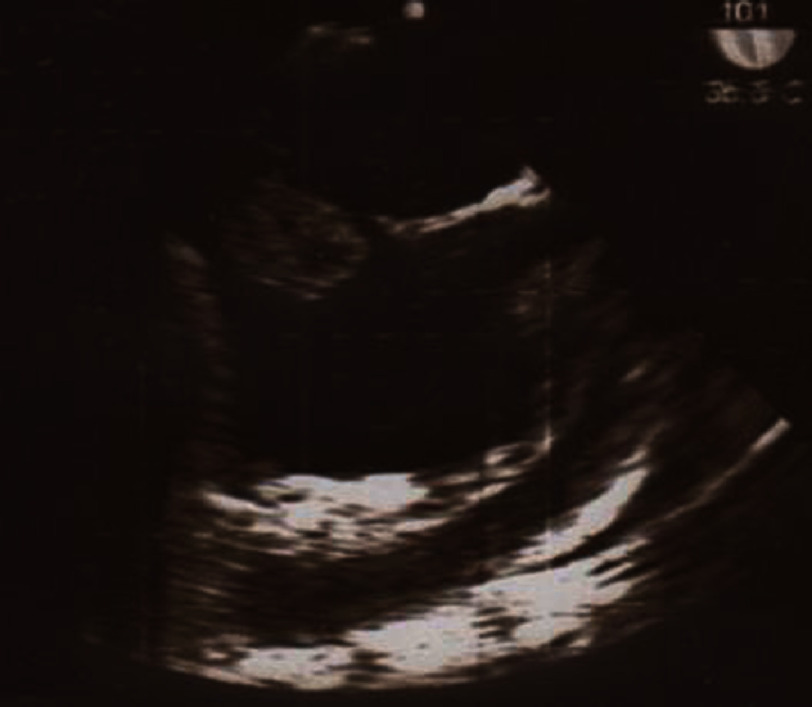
Transesophageal echocardiography showing thrombosis in opening of inferior vena cava.
